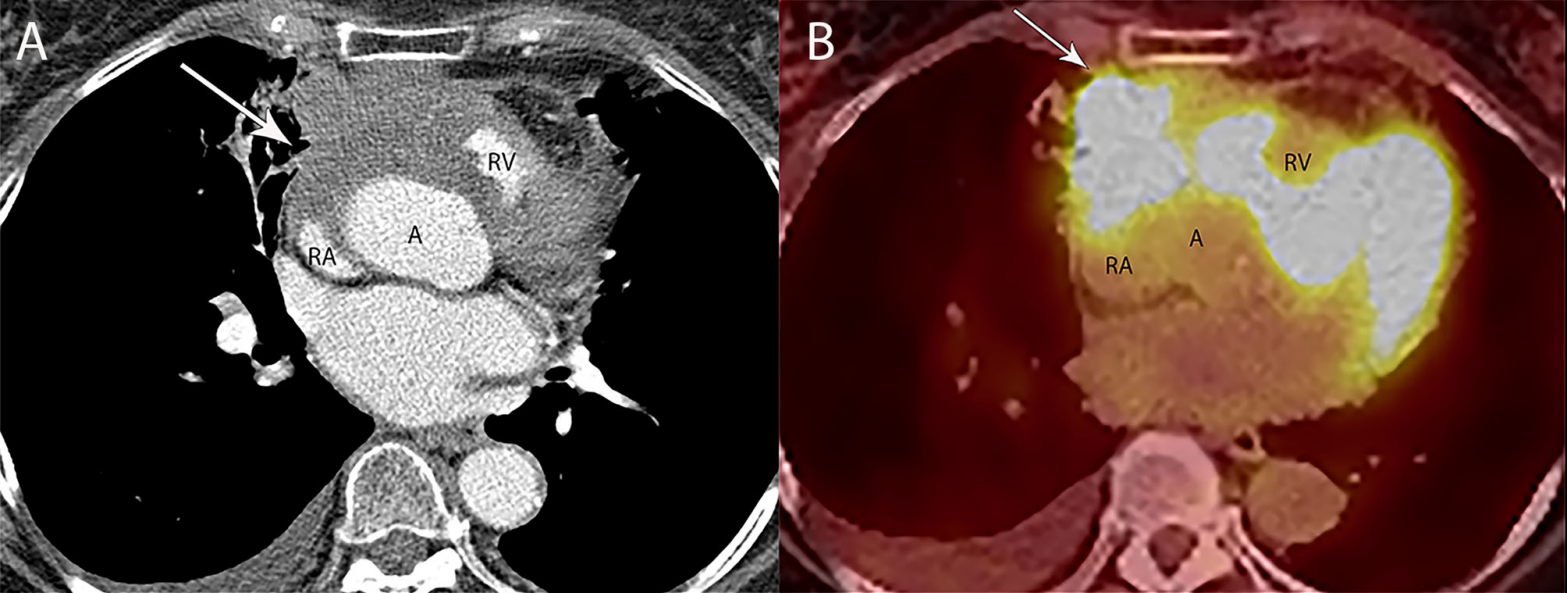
80 year old woman with adenosquamous thymic carcinoma. (A) Contrast-enhanced CT shows right prevascular mediastinal tumor (arrow) invading the pericardium between the right atrium (RA), ascending aorta (A) and right ventricle (RV). (B) PET/CT shows the tumor is markedly FDG avid.
